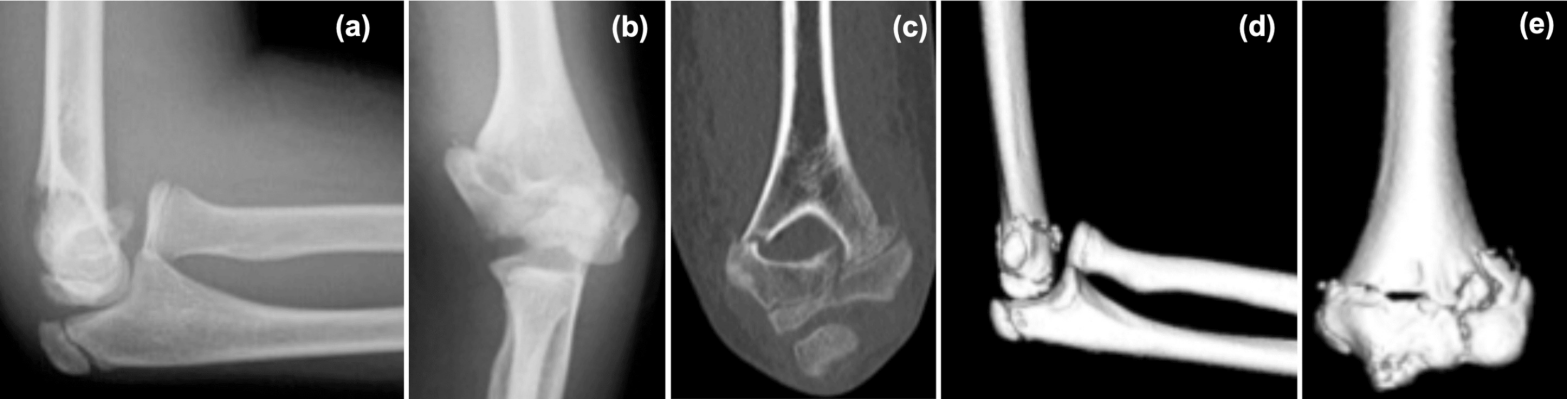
Elbow images on the day of injury (a) X-ray lateral view. (b) X-ray anteroposterior view. (c-e) Computed tomography images suggested a fracture line in the humeral condyle, consistent with a pediatric T-condylar fracture.
